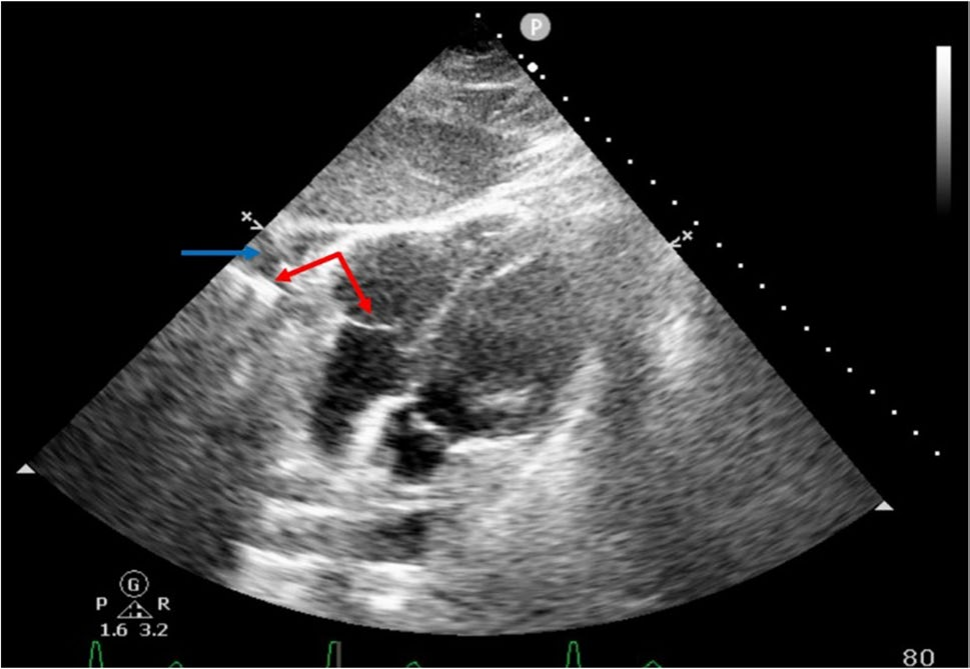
Transthoracic echocardiogram demonstrating an inferior vena cava filter strut traversing the right ventricular free wall (red arrow). This is accompanied by pericardial effusion (blue arrow)
